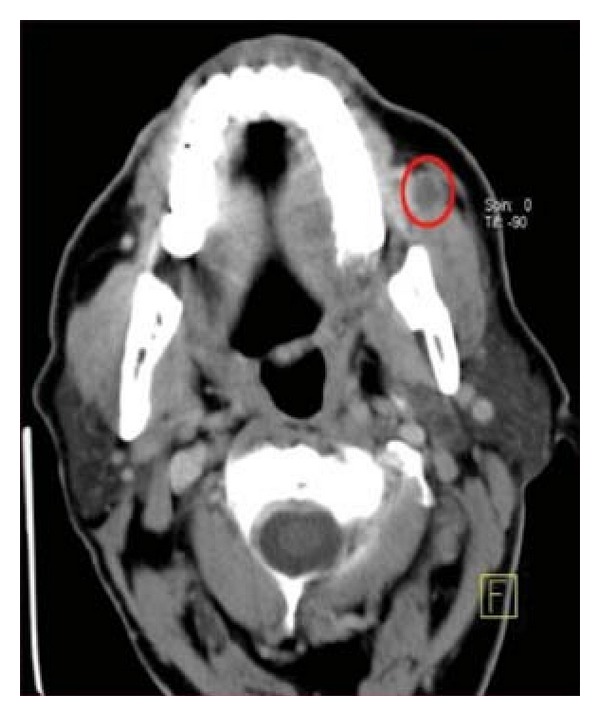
Head CT image showing a malignant lesion in the left buccal mucosa.
